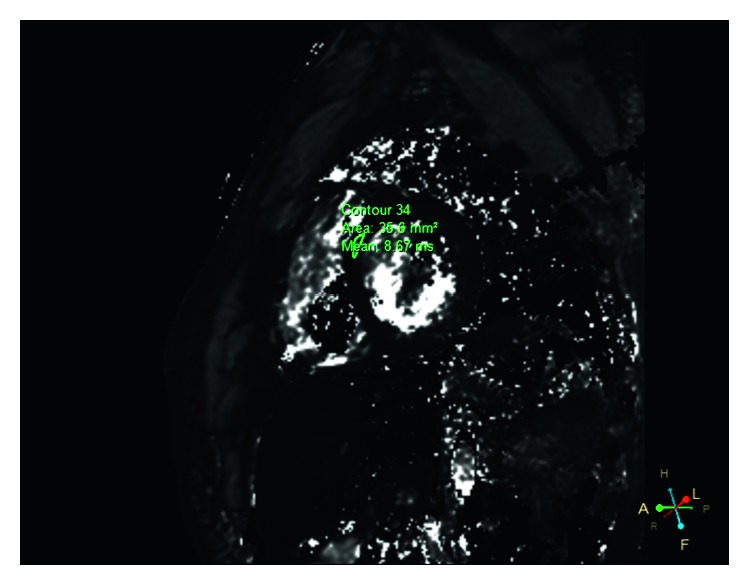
Cardiac magnetic resonance imaging (MRI) finding: T2^*∗*^ mapping MRI sagittal midventricular view shows a dark appearance of left ventricle myocardium due to severe iron load (T2^*∗*^ value was 8.67 ms).
